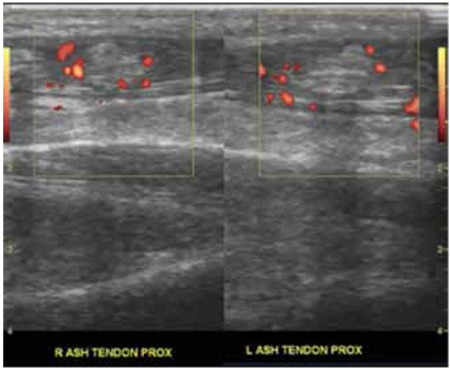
Calcific nodules and surrounding increased Doppler signals localized in both Achilles tendons on the longitudinal Doppler sections
